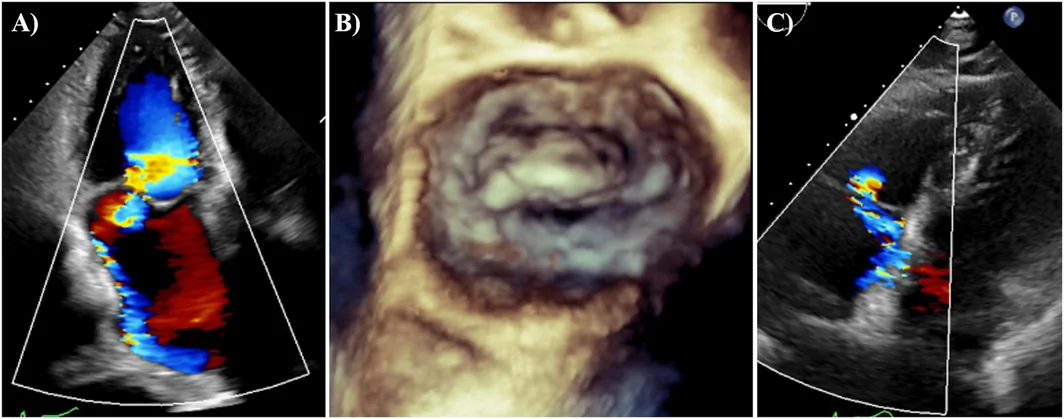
Transesophageal echocardiography (TEE) displaying severe mitral regurgitation (A). Three‐dimensional TEE demonstrating the prolapse of the A1‐2 segments (B). TEE revealing moderate tricuspid regurgitation (C).
